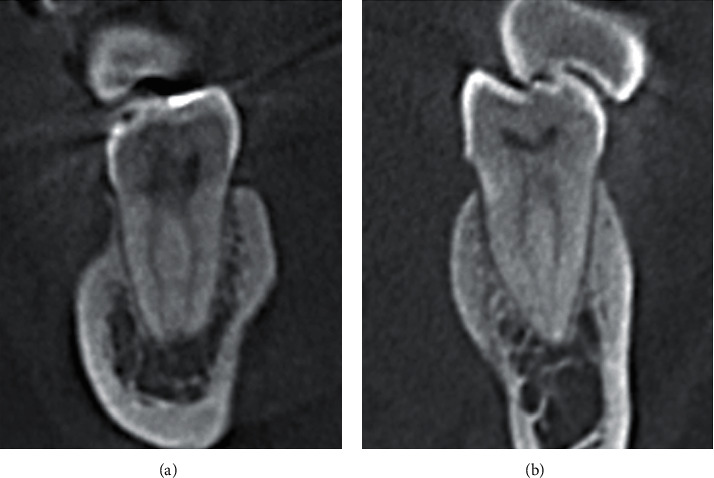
CBCT coronal sections. (a) Vertucci type IV canal configuration in mesial root of a mandibular first molar and (b) Vertucci type II in distal root of a mandibular first molar.
